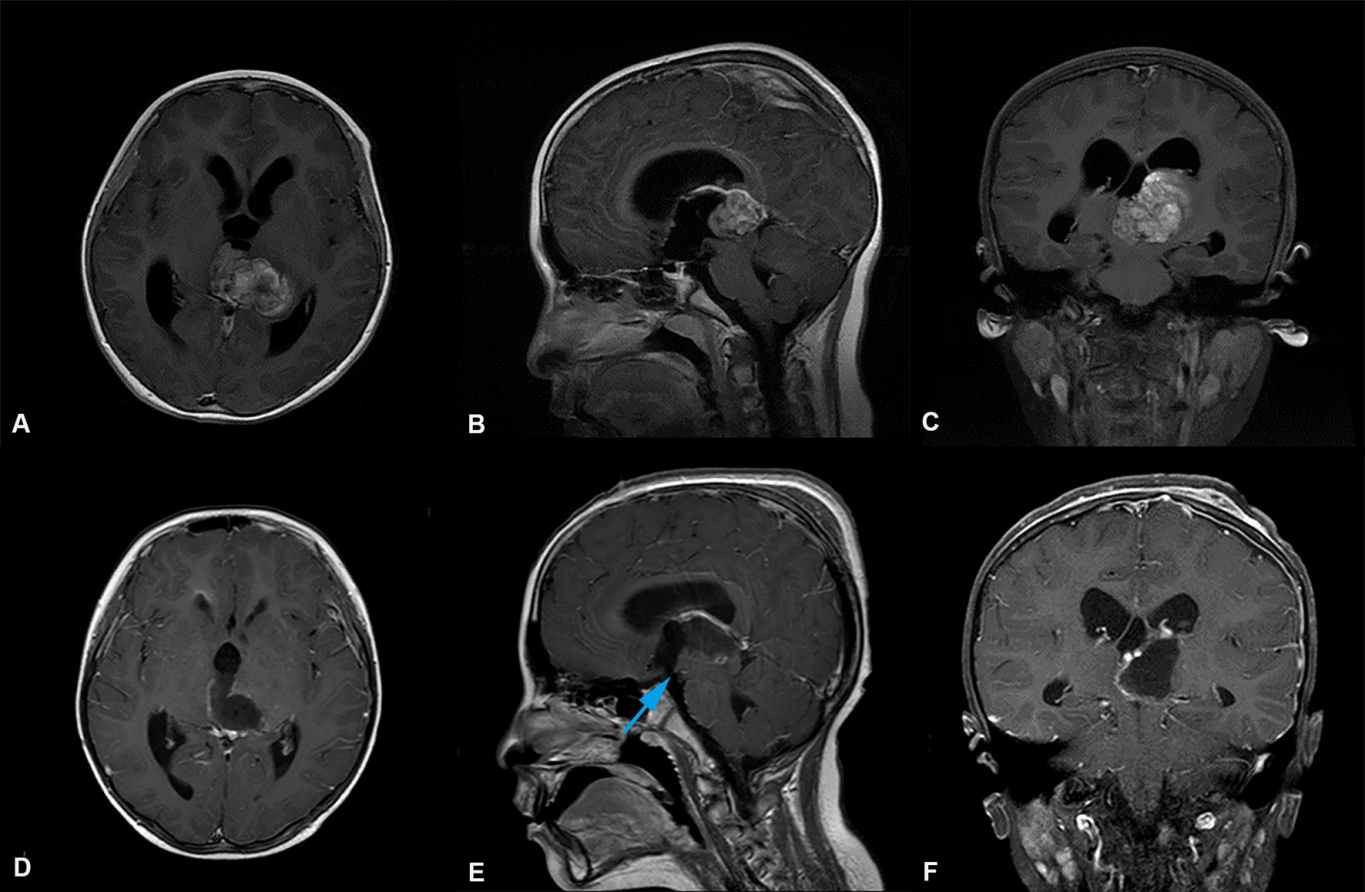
Preoperative and postoperative imaging of thalamic malignant tumor resection with left trans-frontal lateral ventricle approach + third ventriculostomy. (A-C) display: Axial, sagittal and coronal enhanced MRI of malignant tumors of the left thalamus. MRI shows that the main body of the tumor was located in the left thalamus, invading the third ventricle, the right thalamus, the supratentorial lateral ventricle, and the expansion of the third ventricle. (D-F) display: MRI of thalamic tumor resection with left trans-frontal lateral ventricle approach + third ventriculostomy. The images show that the tumor was completely removed, and the volume of the lateral ventricle did not increase and expand. The position indicated by the blue arrow (
